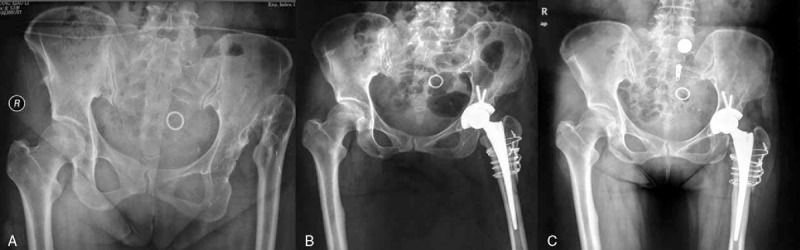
The preoperative (A), immediate postoperative (B), and 1-year (C) radiographic results in a 53-year-old woman with high congenital hip dislocation underwent a cementless total hip arthroplasty using treated with extended sliding trochanteric osteotomy.
